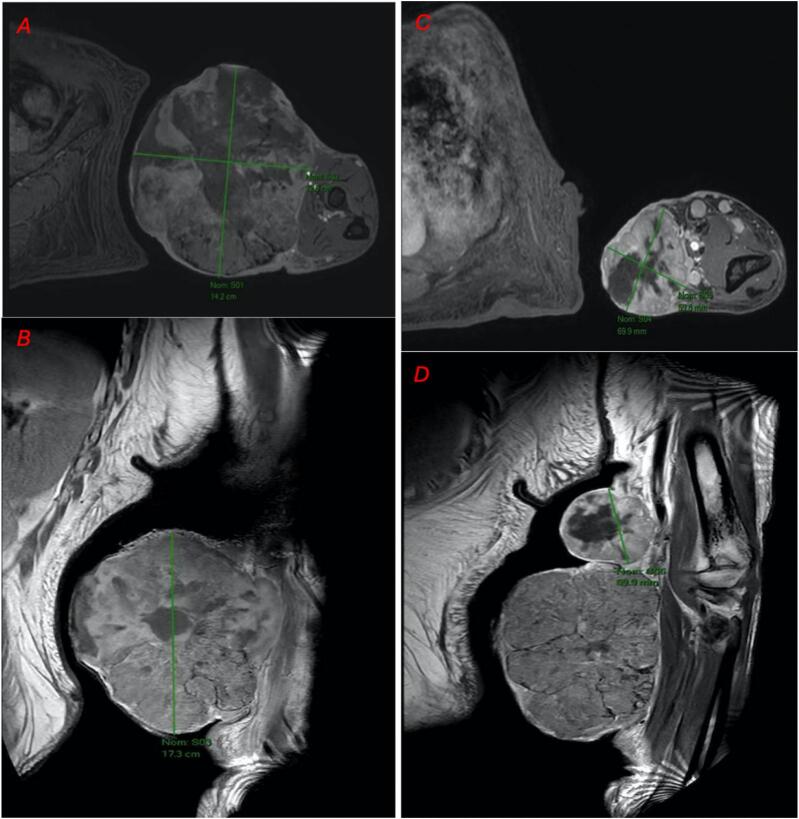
Magnetic Resonance Imaging revealed the two large masses on the left forearm. First large mass measuring 16 × 13 cm in T1 ponderation (A) and in T2 ponderation (B). Second mass measuring 7 cm × 8 cm in T1 ponderation (C) and in T2 ponderation (D).
